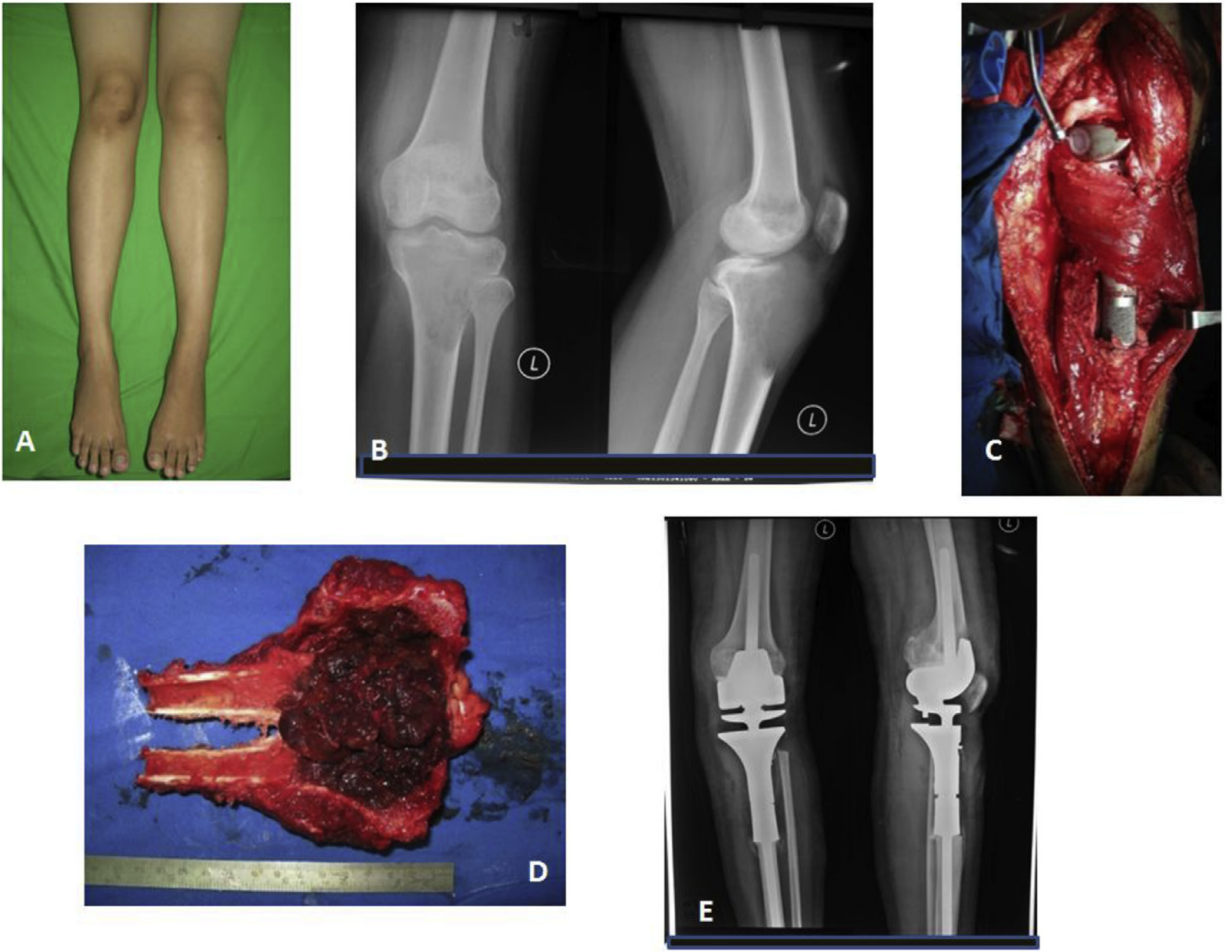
En bloc resection and reconstruction with megaprosthesis of the GCT at proximal tibia. A). Clinical picture of the GCT at the left proximal tibia;B).Radiograph of the patient before surgery; C). Reconstruction of the bone defect with proximal tibia megaprosthesis and medial gatrocnemius flap;D).Gross pathology of the GCT ;E). Postoperative radiograph with megaprosthesis
